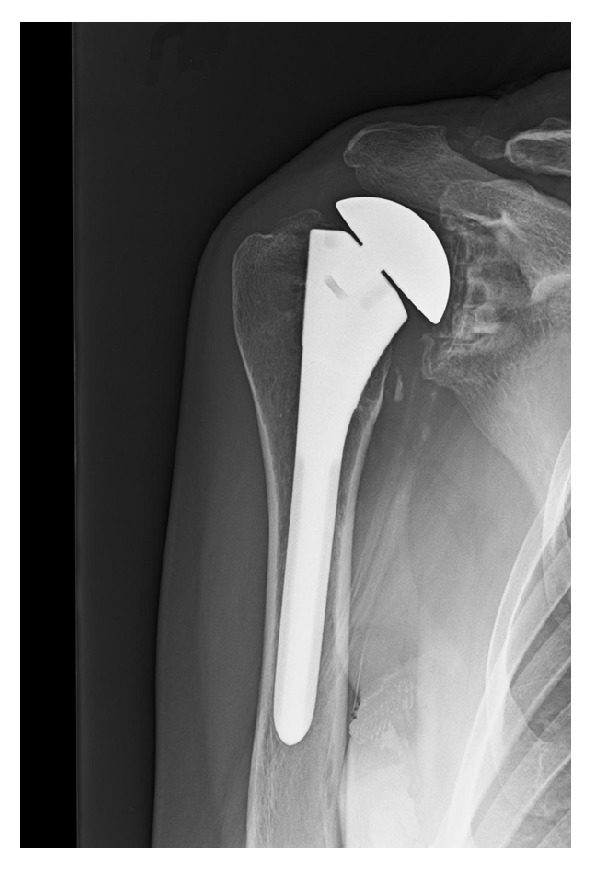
Anterior-posterior radiograph of the patient's right shoulder taken eight months after the revision of the humeral head (16 months after the index TSA). Narrowing of the distance between the acromion and the humeral head seen in this image is suggestive of weakness or a tear of the superior rotator cuff [28].
